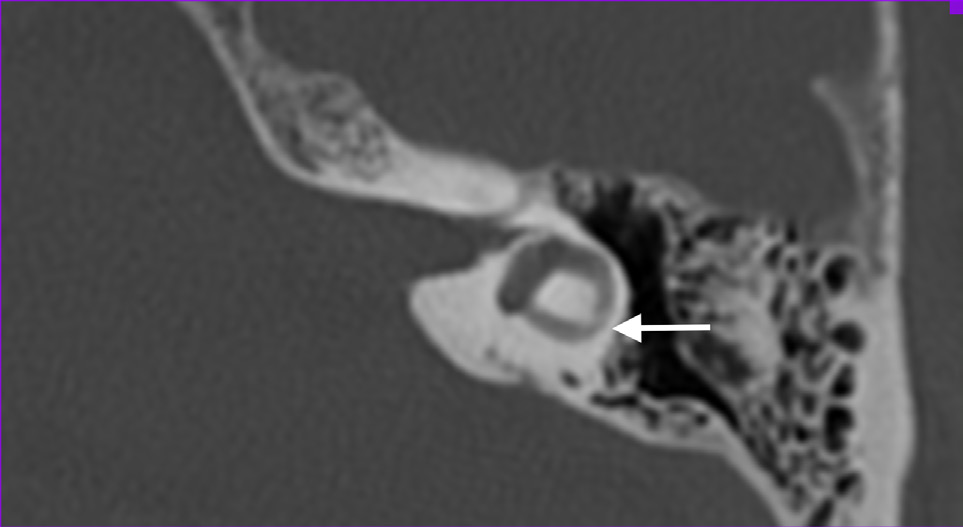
Axial temporal bone CT-scan of the left labyrinth demonstrating narrowing of the lateral semicircular canal (arrow).
